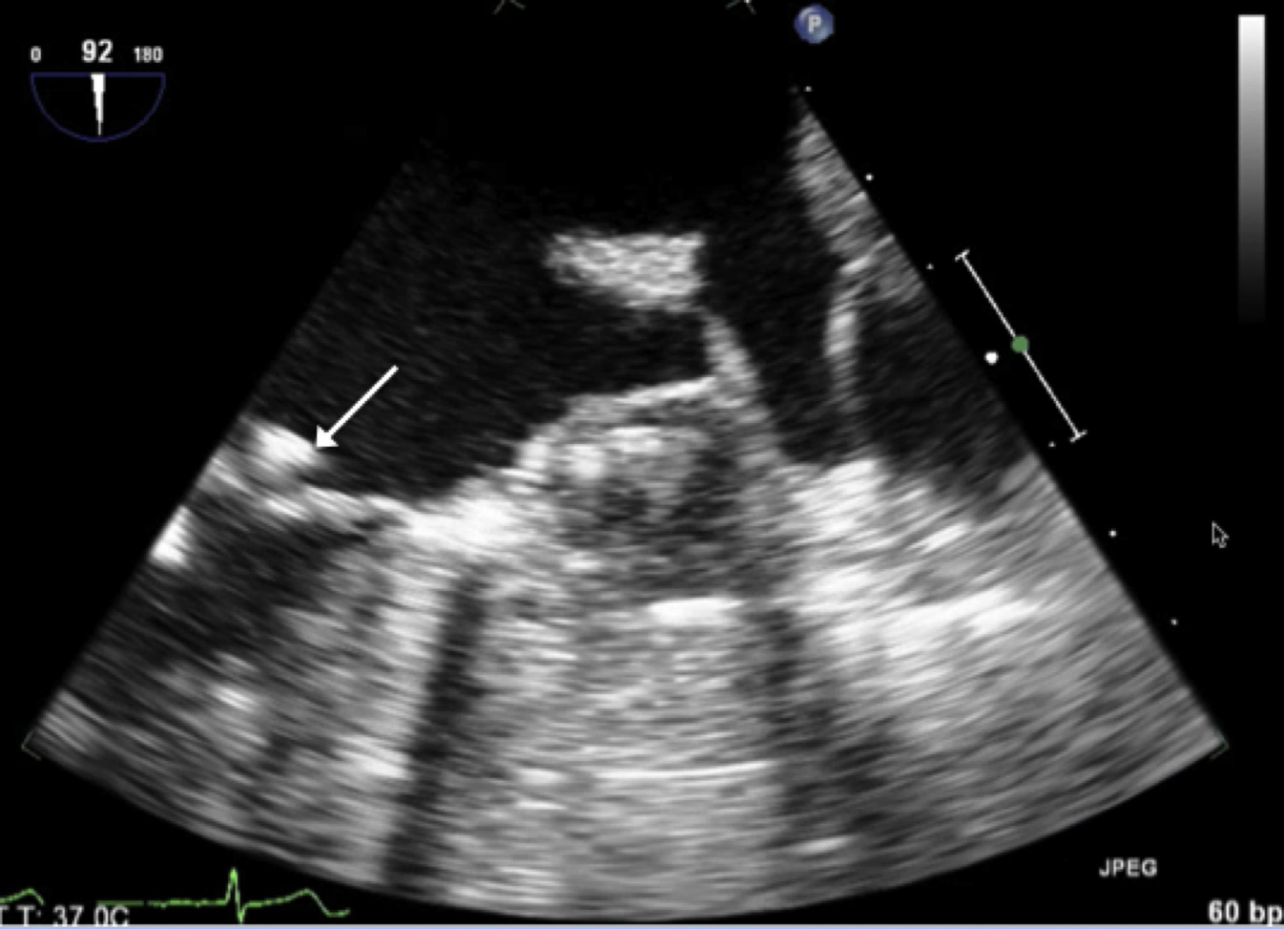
A 45-day post-procedural transesophageal echocardiogram (TEE) image demonstrating the mitral valve mass and WATCHMAN (Boston Scientific, Marlborough, MA) device. TEE image at 90^o^ confirming a stable mitral valve mass (*white arrow*) and endothelialized WATCHMAN device.
